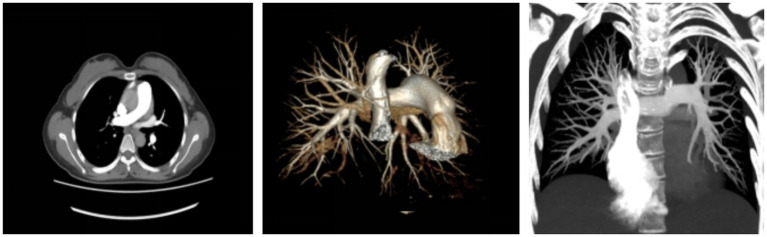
Pulmonary artery CTA findings of the patient on postpartum day 24. Demonstrating well-visualized bilateral pulmonary artery branches, with emboli in partial branches of the left pulmonary artery essentially absorbed.
